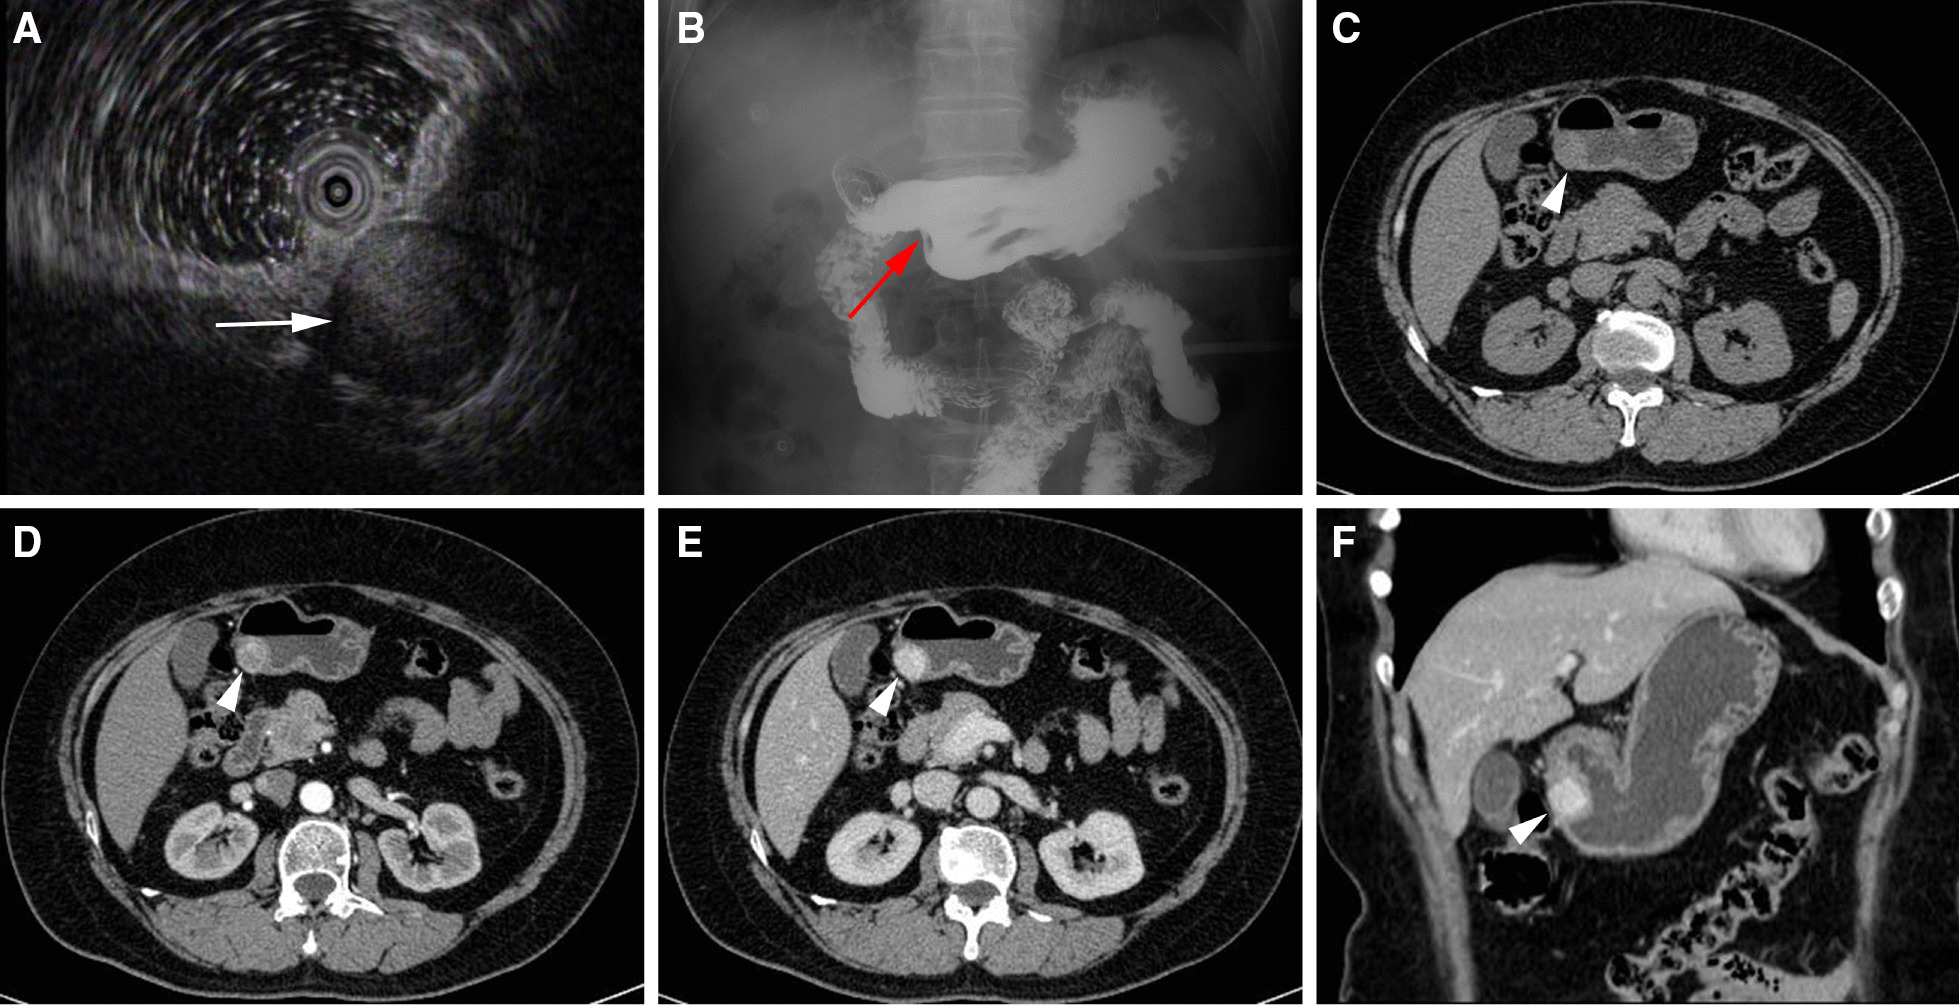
Images of benign GGT located on the greater curvature of gastric antrum. A Endoscopic ultrasonography shows hypoechoic lesion with local heterogenous echo (white arrow), which originates from muscularis propria. B Upper gastrointestinal barium meal radiography shows a round filling defect with smooth boundary in the greater curvature of gastric antrum (red arrow). C Non-contrasted CT shows round soft tissue density in the lesser curvature of the gastric antrum (arrowhead), the average CT value was about 41 Hu. D Contrasted CT shows obvious enhancement in arterial phase, the average CT value was about 92 Hu. E Portal venous phase image shows central filling enhancement, the average CT value was about 140 Hu. F The contrast enhancement coronal image in the portal phase shows homogenous density of the lesion with well-defined boundary
